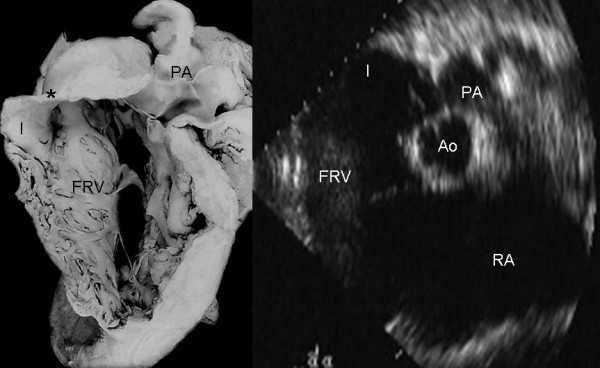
Internal view of the functional portion of the right ventricle shows significant dilatation of the infundibulum (I) with thinning of its wall (Asterisk). The echocardiographic image at the level of great vessels shows dilatation of the infundibulum (I). Abbreviations as before.
